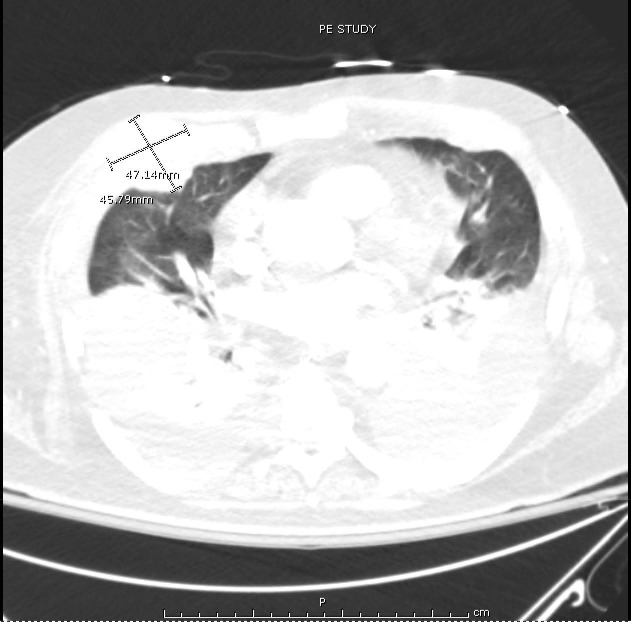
Computed tomography (CT) of chest showing widespread lobulated masses present bilaterally, largest measuring 4.6 x 4.7 cm in the right mid chest with bilateral pleural effusions causing severe compressive atelectasis
